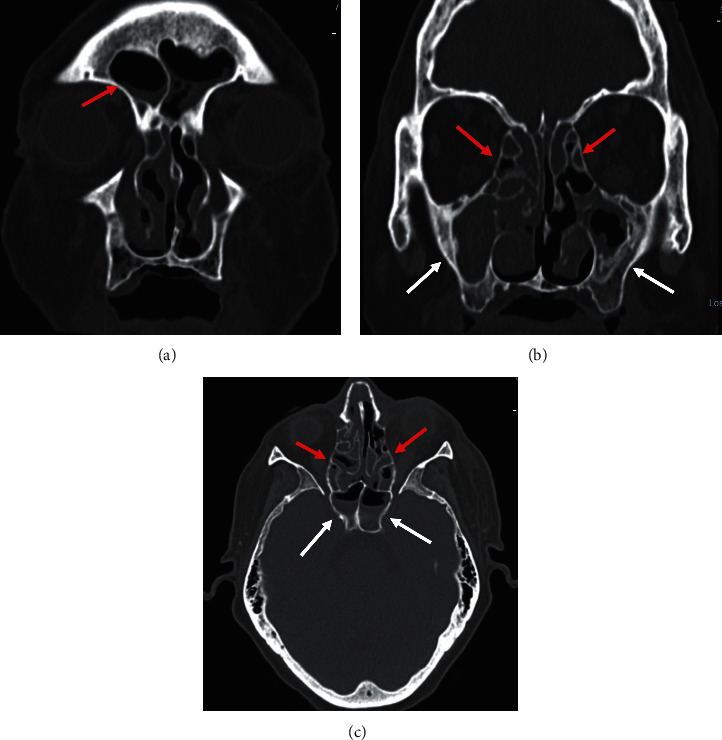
(a) Paranasal sinuses CT scan showed fluid level of frontal sinuses, (b) mucosal thickening of bilateral maxillary (white arrows) and ethmoid sinuses (red arrows), and (c) mucosal thickening of bilateral ethmoid sinuses (red arrows) and fluid level of bilateral sphenoidal sinuses (white arrows).
